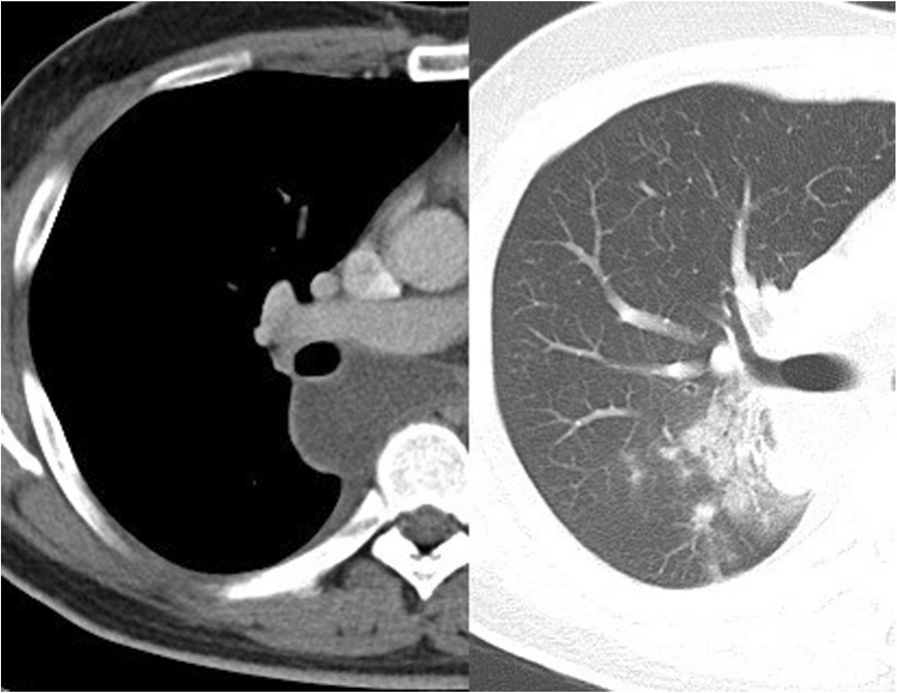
Enhanced computed tomography scan at our hospital showing a reduction in the tumor and right intrapulmonary consolidation
